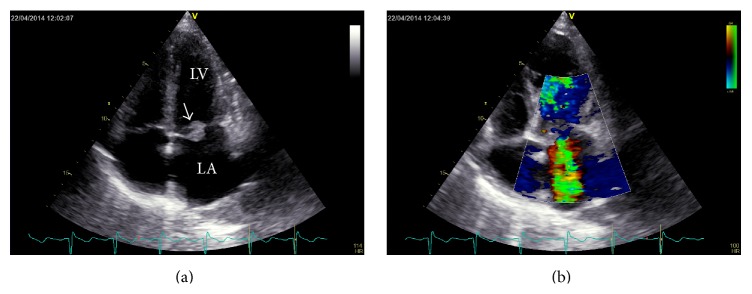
Transthoracic echocardiography (TTE), 4-chamber view of the heart. (a) The mitral valve is thickened and dysfunctional due to a floating vegetation (white arrow) on the anterior mitral leaflet; LA = left atrium; LV = left ventricle. (b) Color Doppler sonography shows severe mitral regurgitation.
